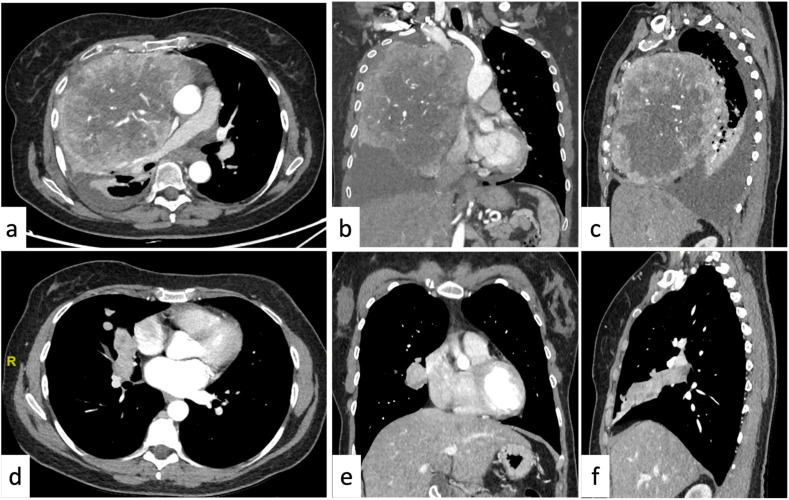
Mediastinal neuroendocrine tumor (NET) vs. lung carcinoid. (a–c) Mediastinal neuroendocrine tumor (NET). 40-year-old male patient with a sensation of chest weight and dyspnea. Contrast-enhanced axial CT scans (a) with coronal (b) and sagittal (c) reconstruction show a voluminous vascularized solid mass, markedly inhomogeneous for the presence of necrotic tissue, occupying most of the right hemithorax, probably originating from the anterior mediastinum; it determines dislocation, compression, and infiltration of the vascular structures of the mediastinum, thus like pleural planes. Ipsilateral pleural effusion and overlying pulmonary parenchyma atelectasis are associated. (d–f) Similar CT features are seen in a 44-year-old female. Contrast-enhanced CT (d–f): it shows a solid nodule in the right ilo-parahilar site of the middle lobe, with inhomogeneous post-contrastographic enhancement. This lesion appears in proximity to the lateral wall of the right atrium (without clear signs of infiltration). These characteristics are suspicious for malignant nature; the biopsy confirmed the diagnosis of typical pulmonary carcinoid.
